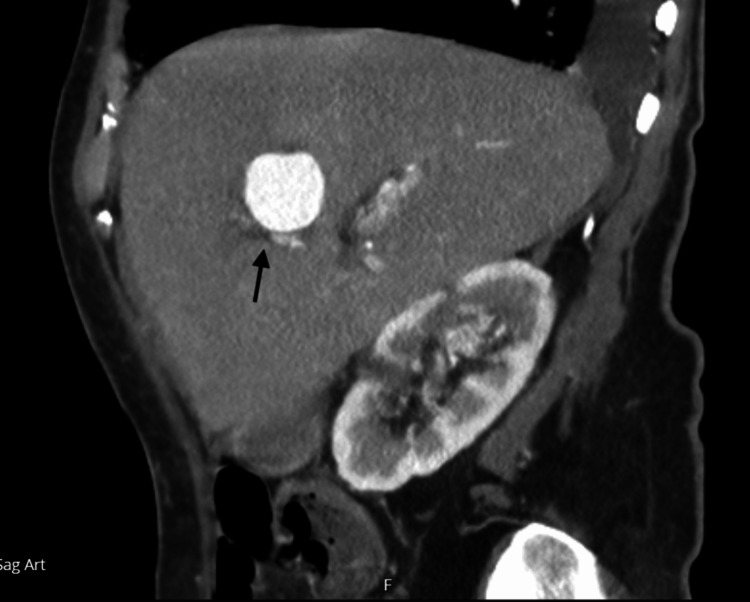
Triple phasic Computed Tomography of the liver in the sagittal view demonstrating a 3 cm x 2.5 cm aneurysm (black arrow) arising from a branch of the left hepatic
